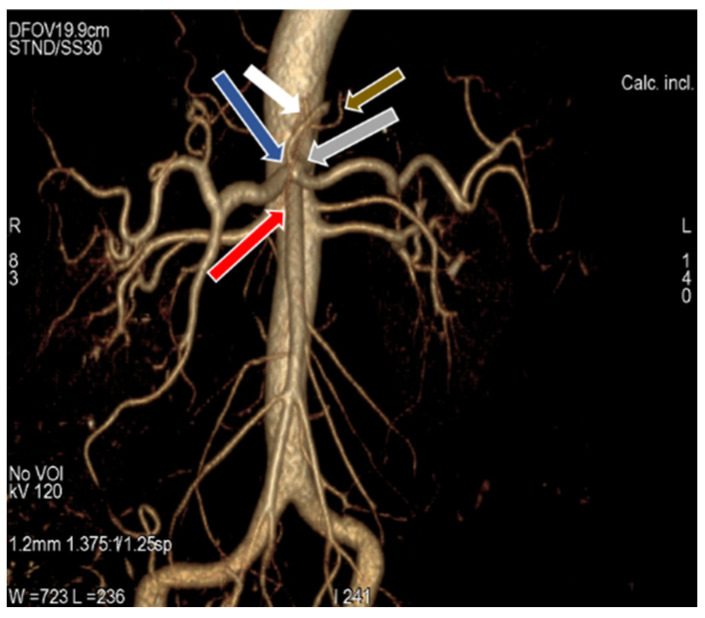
3D-VR image of (64 row scanner CT angiography) abdominal region showing the Celio-mesenteric trunk (grey arrow), which divided into celiac trunk (blue arrow) and superior mesenteric artery (red arrow). Left gastric artery gives rise to right inferior phrenic artery (brown arrow) and left inferior phrenic artery (white arrow).
